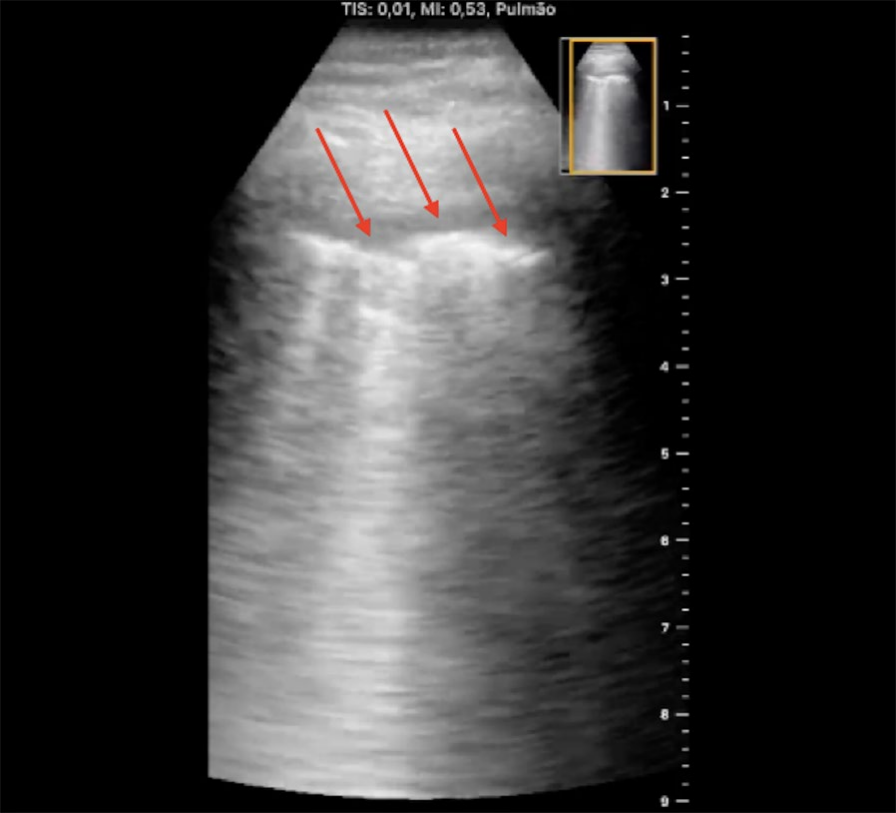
Sonographic appearance of pleural irregularities in a COVID-19 patient. Red arrows indicate fragmented and irregular pleural line
